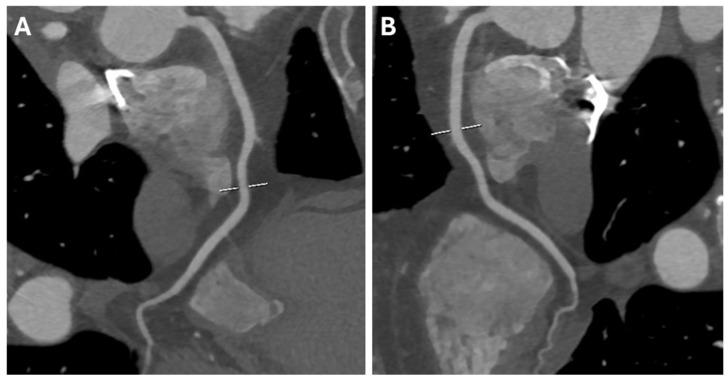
Computed tomography coronary angiography of the same patient discussed in Figure 2, showing a non-obstructed left anterior descending artery (A) and right coronary artery (B) after an episode of chest pain with troponin elevation.
